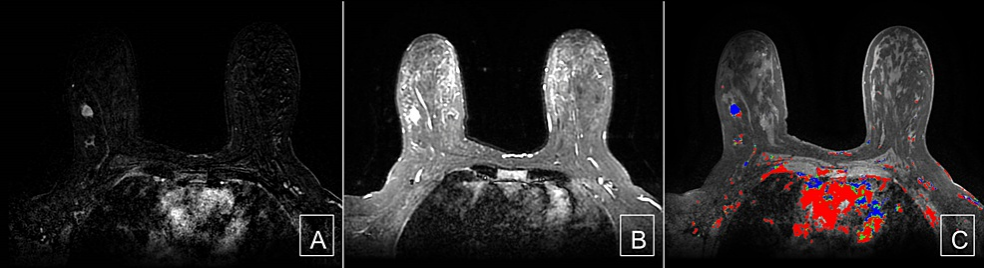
Hemangioma: subtracted image, T2 image, and kinetics image (A) A fat-saturated subtracted T1 weighted post-contrast image demonstrates irregular mass with irregular margins with heterogeneous internal enhancement. (B) A fat-saturated T2-weighted image demonstrates a high signal within the capillary hemangioma. (C) A fat-saturated T1-weighted post-contrast with kinetic overlay demonstrates predominantly persistent washout kinetics.
